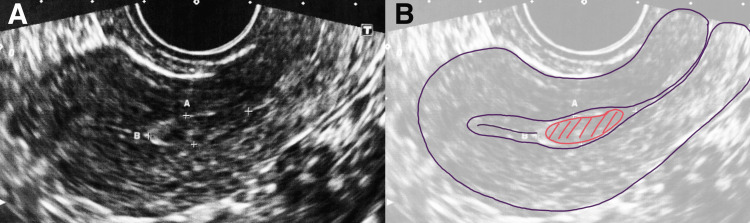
Preoperative transvaginal ultrasound A: Preoperative transvaginal ultrasound revealed that the polyp had already become indistinct and significantly reduced in size. B: Schermer depicts the endometrial polyps located on the lower segment of the uterine body.
